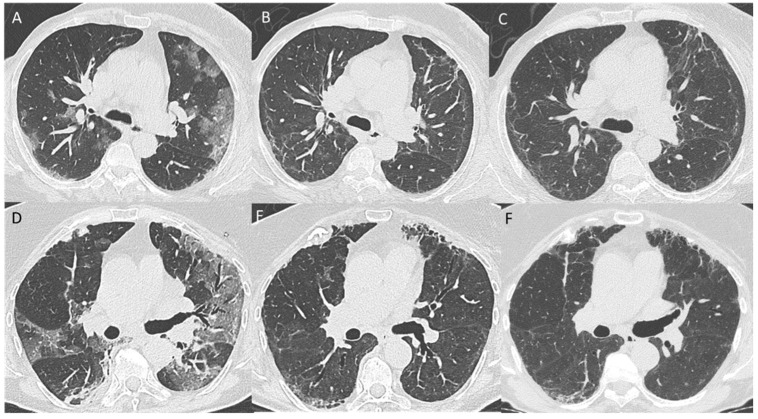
Baseline (A), 6-month follow-up (B), and 12-month follow-up (C) axial CT images showing the evolution of organizing pneumonia (OP) features towards residual non-fibrotic abnormalities resembling NSIP. Baseline (D), 6-month follow up (E), and 12-month follow-up (F) axial CT scans, showing patchy ground glass opacities (GGO) that are progressively replaced by reticular abnormalities and mild traction bronchiectasis resembling a fibrotic NSIP pattern.
